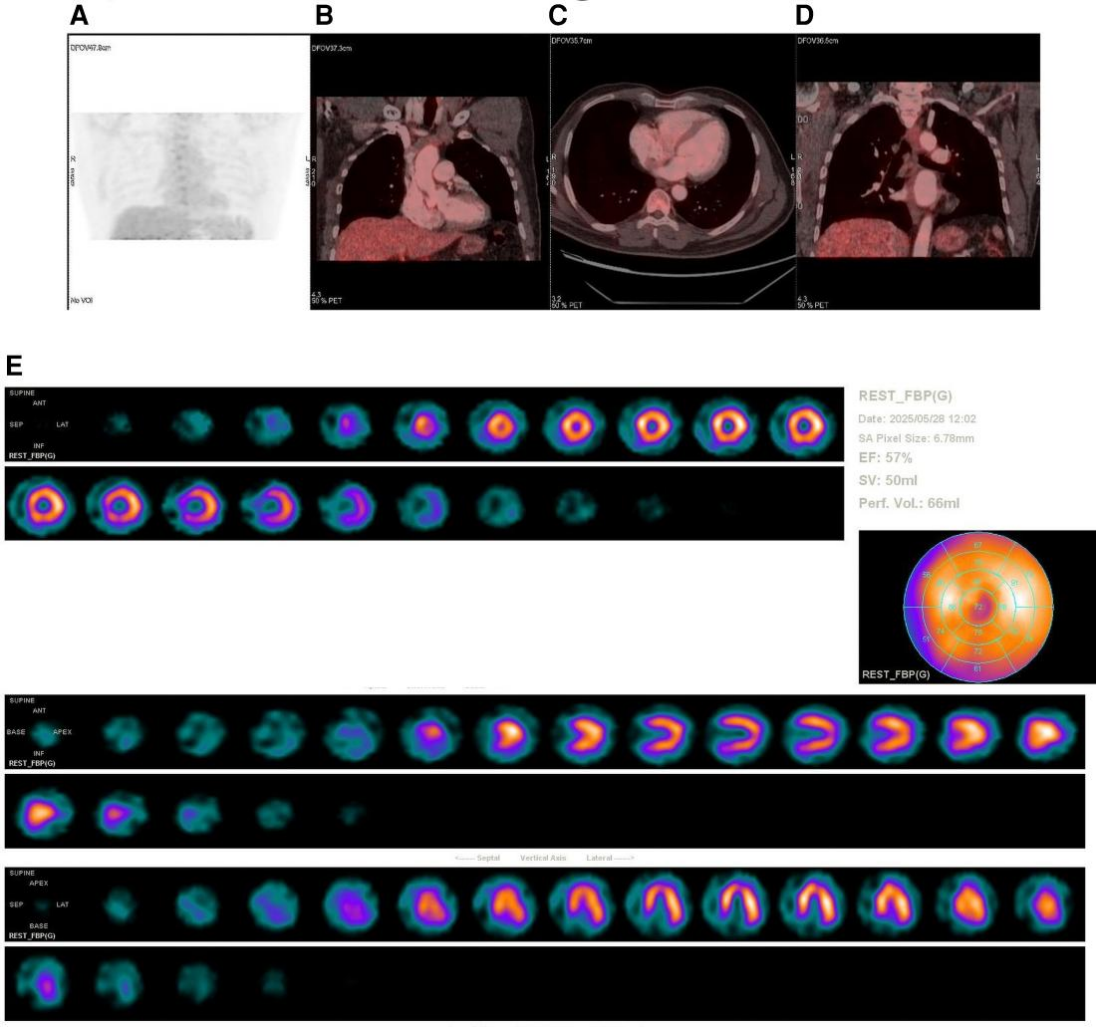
Nuclear medicine imaging. (*A*) Maximum-intensity projection (MIP) of cardiac 18F-FDG PET/CT after a myocardial-suppression protocol shows no focal or diffuse myocardial FDG uptake. (*B–C*) Fused PET/CT coronal and axial images with the heart in the field of view demonstrate physiologic myocardial FDG suppression. (*D*) Fused PET/CT coronal image focused on the mediastinum shows no FDG-avid lymphadenopathy. (*E*) Rest 99mTc-sestamibi myocardial perfusion scintigraphy (MPS): short-axis (SA), polar map, vertical long-axis (VLA), and horizontal long-axis (HLA) views demonstrate a moderate apical perfusion defect with mild–moderate mid- and basal-inferior defects.
